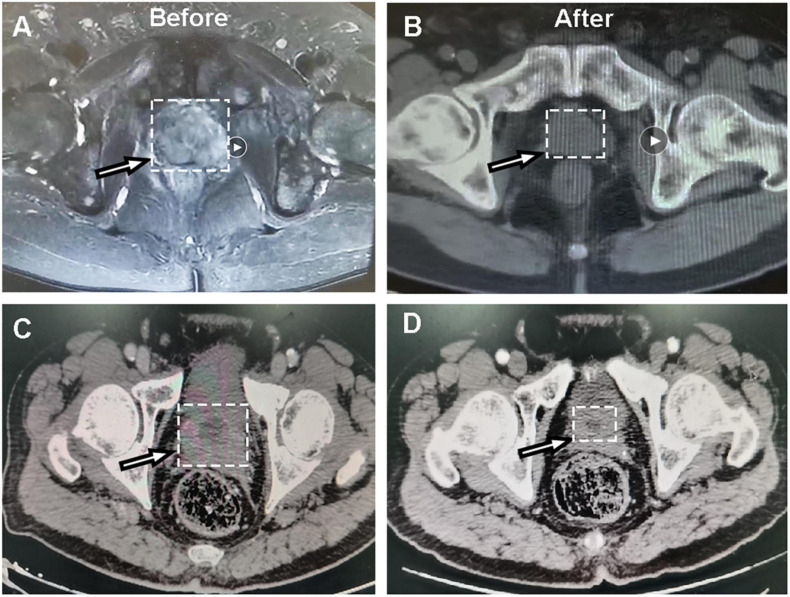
Representative CT images of dicycloplatin-sensitive patients. (A,B) CT images of one patient with complete response taken before (A) and after (B) dicycloplatin treatment. (C,D) CT images of another patient with partial response taken before (C) and after (D) dicycloplatin treatment. White arrow represents the tumor localization.
